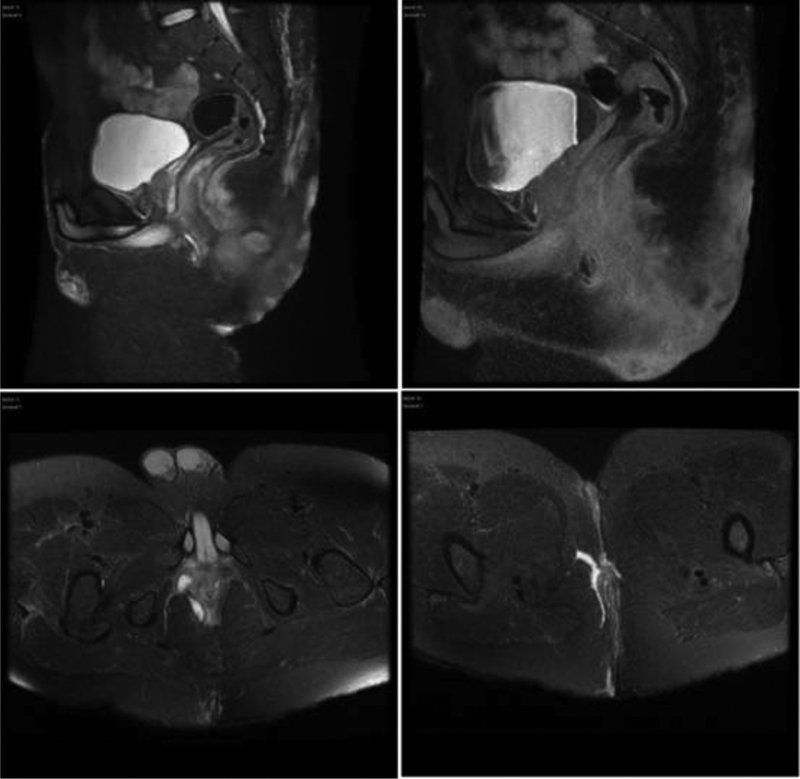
Magnetic resonance imaging of pelvis showing multiple fistulous tract and abscesses.
